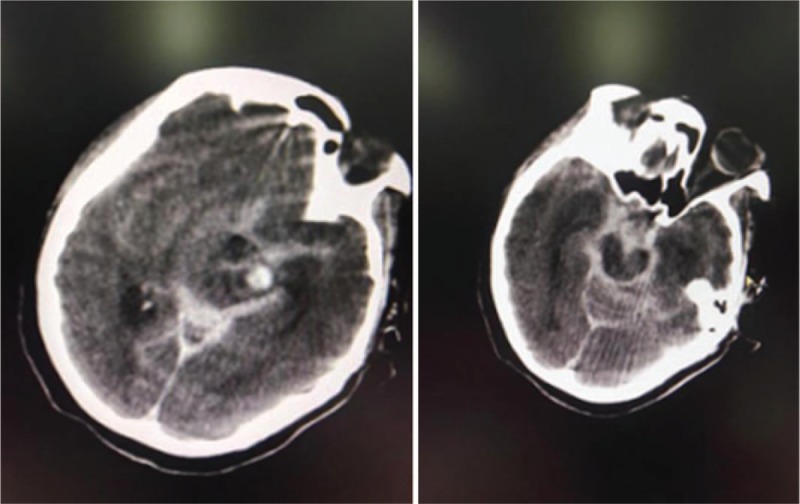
Emergency CT examination of the patient during the cerebral angiography. The images of CT showed ruptured aneurysm. CT = computed tomography.
